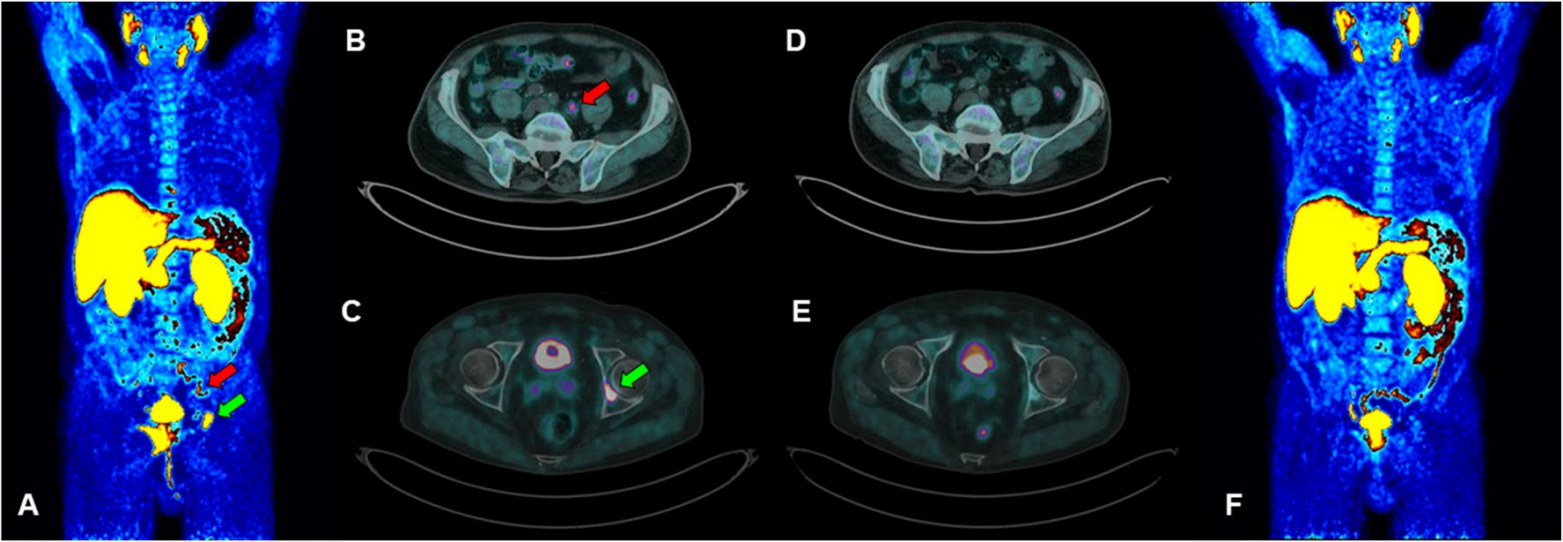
Staging [18F]F-choline PET/CT scan (A maximum intensity projection; B–C transaxial fused images): the exam shows skeletal (green arrows) and lymph node (red arrows) uptakes. A second [18F]F-choline PET/CT scan performed 10 days after the start of ADT (D–E transaxial fused images; F maximum intensity projection) showed almost complete disappearance of pathological uptakes (i.e. SUVmax dicreasing from 33.2 to 3.4 on acetabulum lesion)
